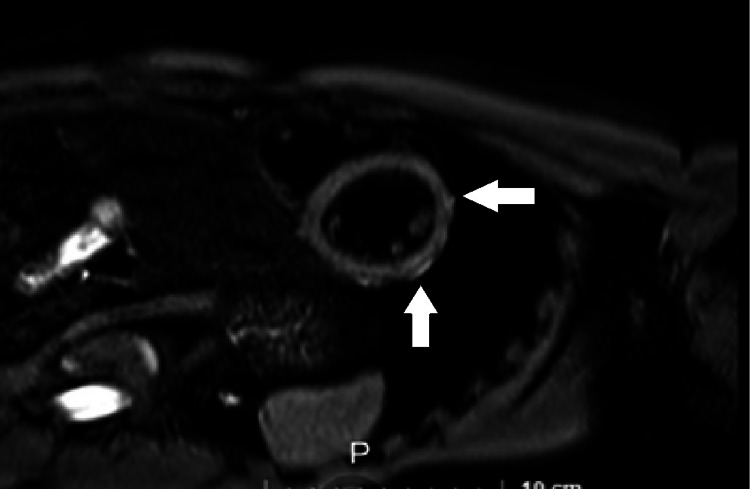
MRI T2-STIR Sequence (2020) Mild global myocardial hyperintensity is observed (arrows)
